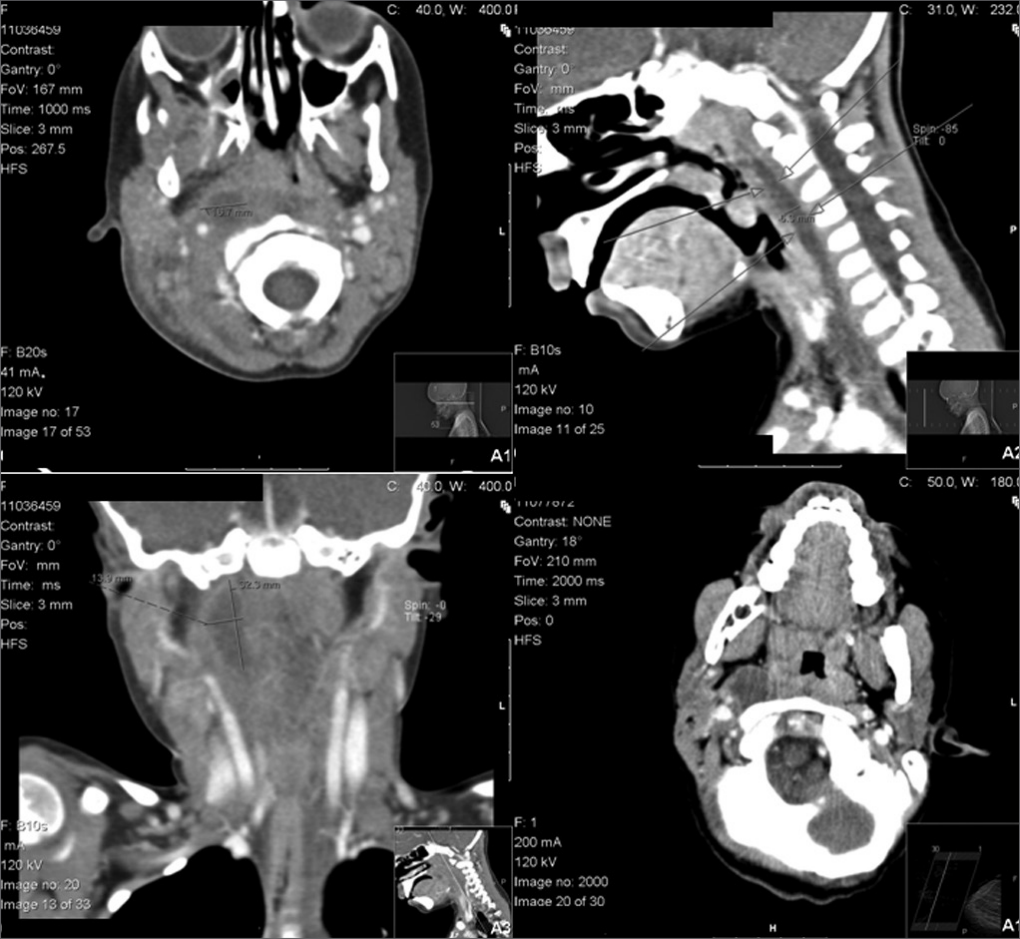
A. Latero and Retropharyngeal abscess (CT scan, axial view). B. Latero and Retropharyngeal abscess (CT scan, sagital view). C. Lateropharygeal abscess (CT scan, coronal view). D. Lateropharyngeal abscess (CT scan, axial view)
